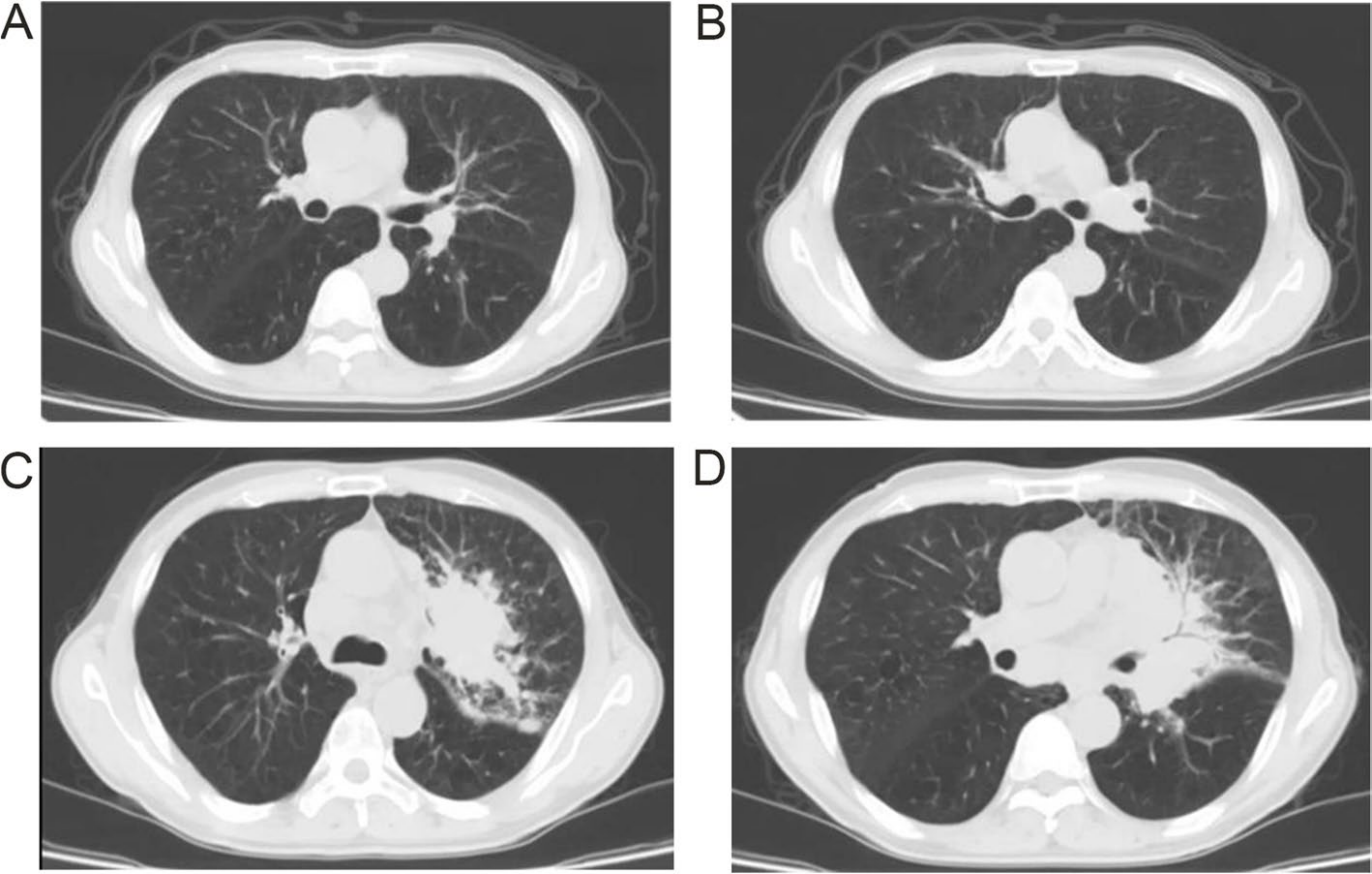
A and B show the CT scan results 2 months after treatment. C and D show the CT scan results after disease progressed
